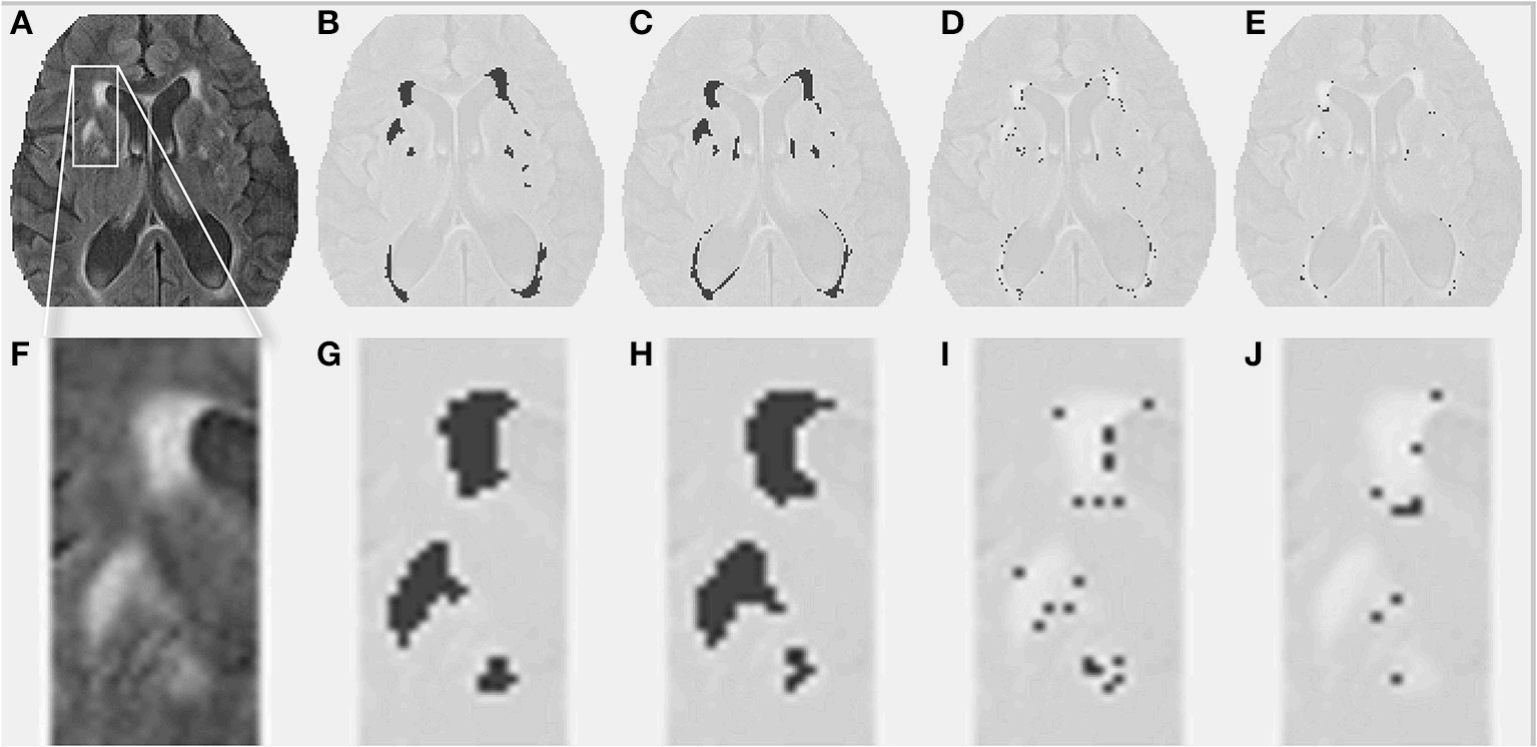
White matter lesions (WML) at a middle level height. (A) FLAIR image for a given subject. (B) Conventionally estimated WML. (C–E) Estimated WML, using the proposed segmentation algorithm, for full, intermediate, and small proportion of lesion. (F–J) Similar images for the zoomed portion depicted by the white box in (A).
